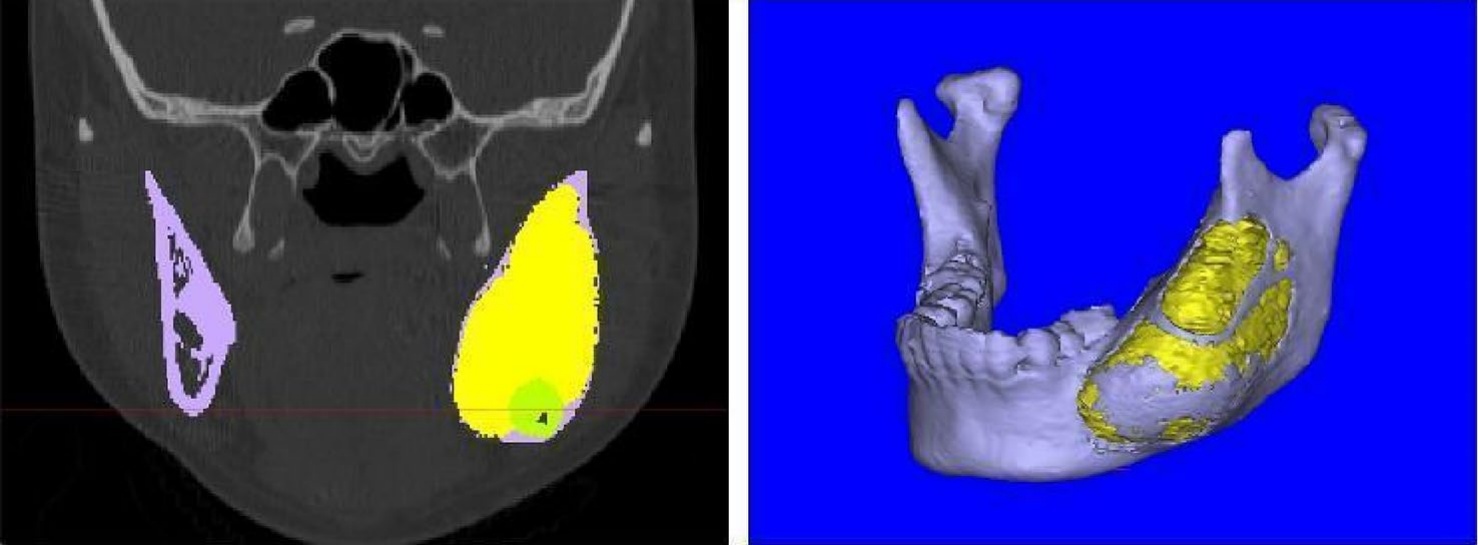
Reconstruction of a 3D model of the mandible, impacted tooth, and lesion. (A) Coronal CT images of the mandible (purple), lesion (yellow), and impacted tooth (green) were selected. (B) A 3D image of the mandible showed severe swelling and resorption of the mandibular ramus and body, with a large defect in the cortical layer.
